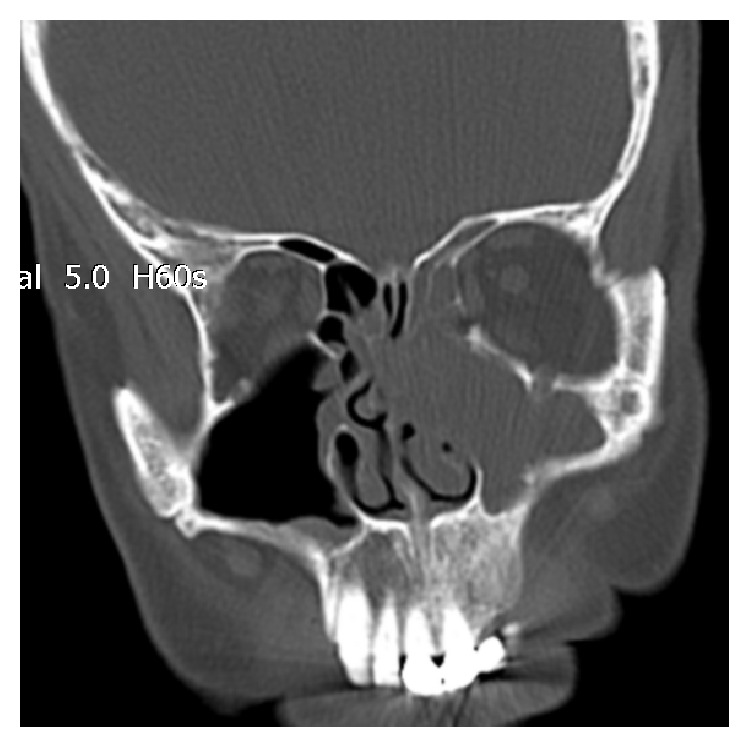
Paranasal sinus computed tomography showing a soft tissue lesion eroding the left maxillary sinus medial wall, filling the ethmoidal cells and frontal sinus and destructing the lamina papyracea.
